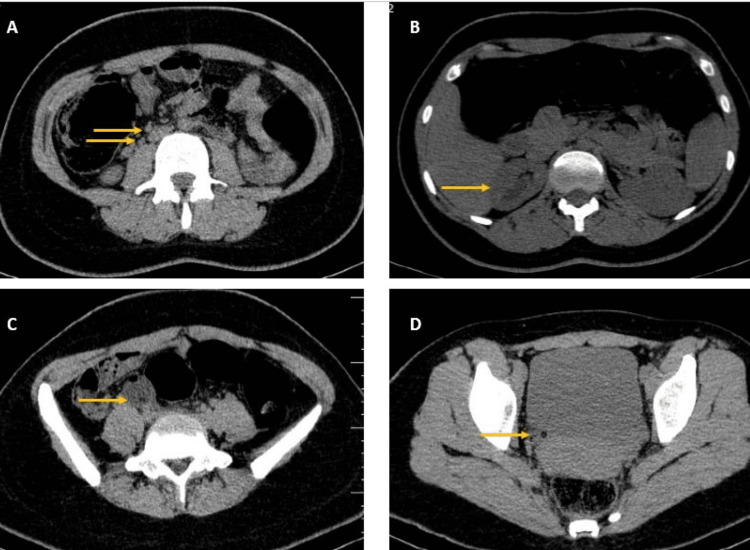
CT scan without contrast findings Duplex ureters (A), mild hydronephrosis of upper moiety (B), Misplaced balloon of Foley catheter in the right lower quadrant (C), and Foley catheter in the ectopic ureter draining to urethra outside of the bladder (D). CT: Computed Tomography
